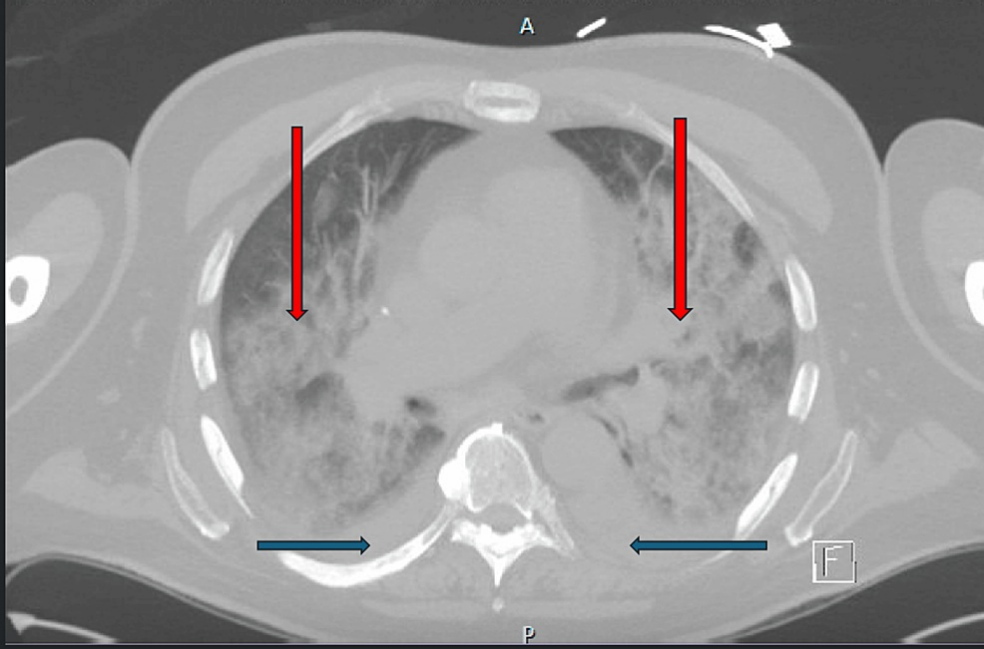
Computed tomography of the chest showing bilateral diffuse ground-glass opacities with small effusions bilaterally Blue arrows: bilateral pleural effusion; Red arrows: bilateral diffuse ground-glass opacities
